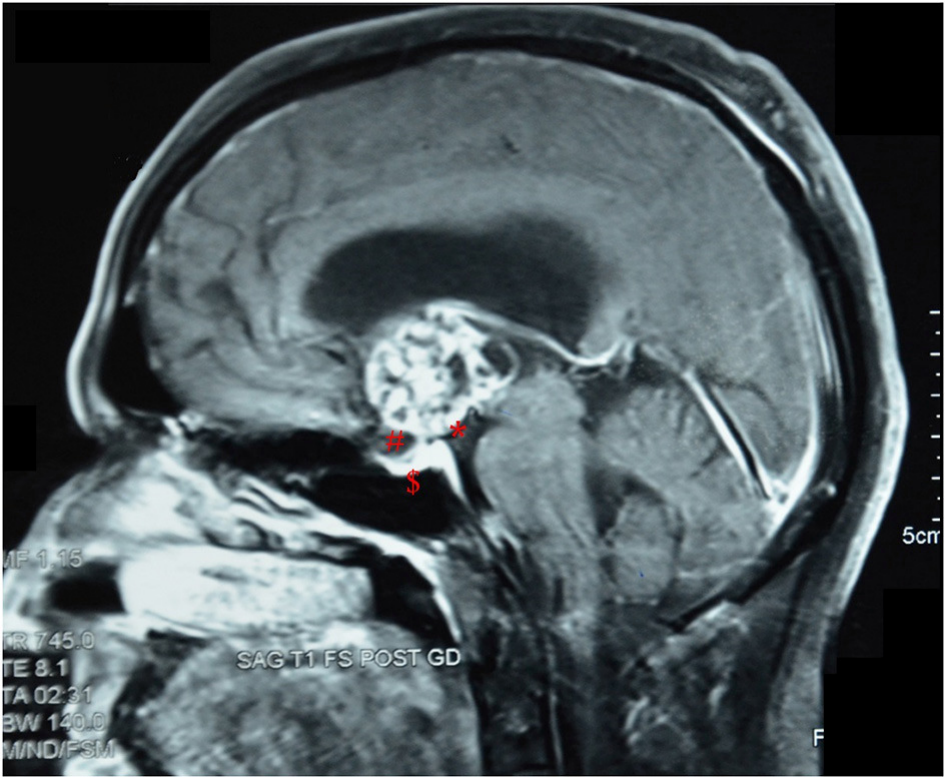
Post-contrast sagittal MRI image of a patient with an intrinsic third ventricular craniopharyngioma, showing features as described by Migliore et al. (16). *, an intact third ventricular floor; #, a patent suprasellar cistern; $, absence of sellar abnormalities.
